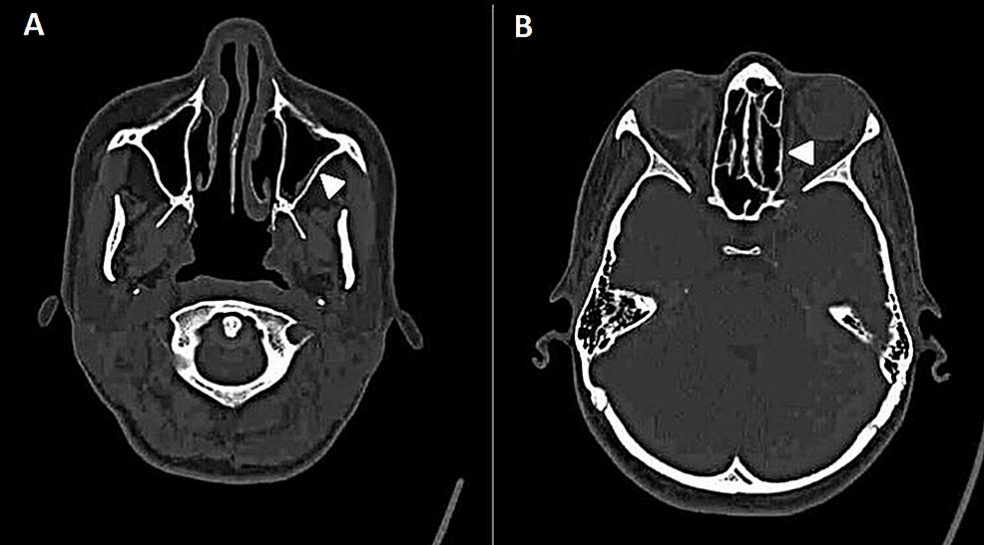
Post-treatment CT scans of the patient. (A) Post-medication total improvement of the opacified left maxillary sinus (arrowhead); (B) improved ethmoid sinuses post-medication use (arrowhead).
